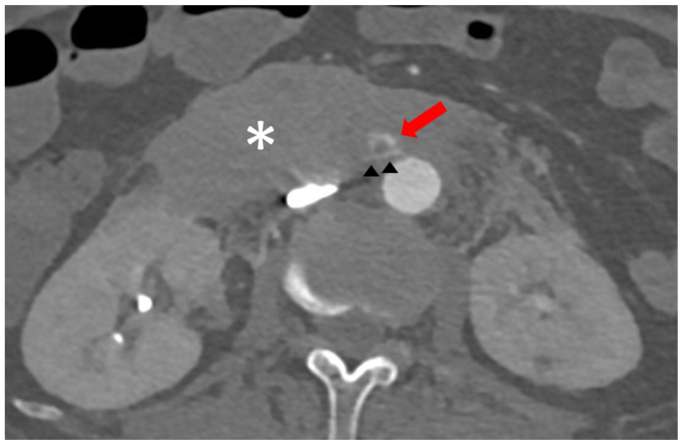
Computed tomography scan showing contrast extravasation near the ostium of the ovarian artery (*red arrow*) with large retroperitoneal hematoma (∗). There was sharp angulation of the right ovarian artery arising from the aorta (*black arrowheads*).
